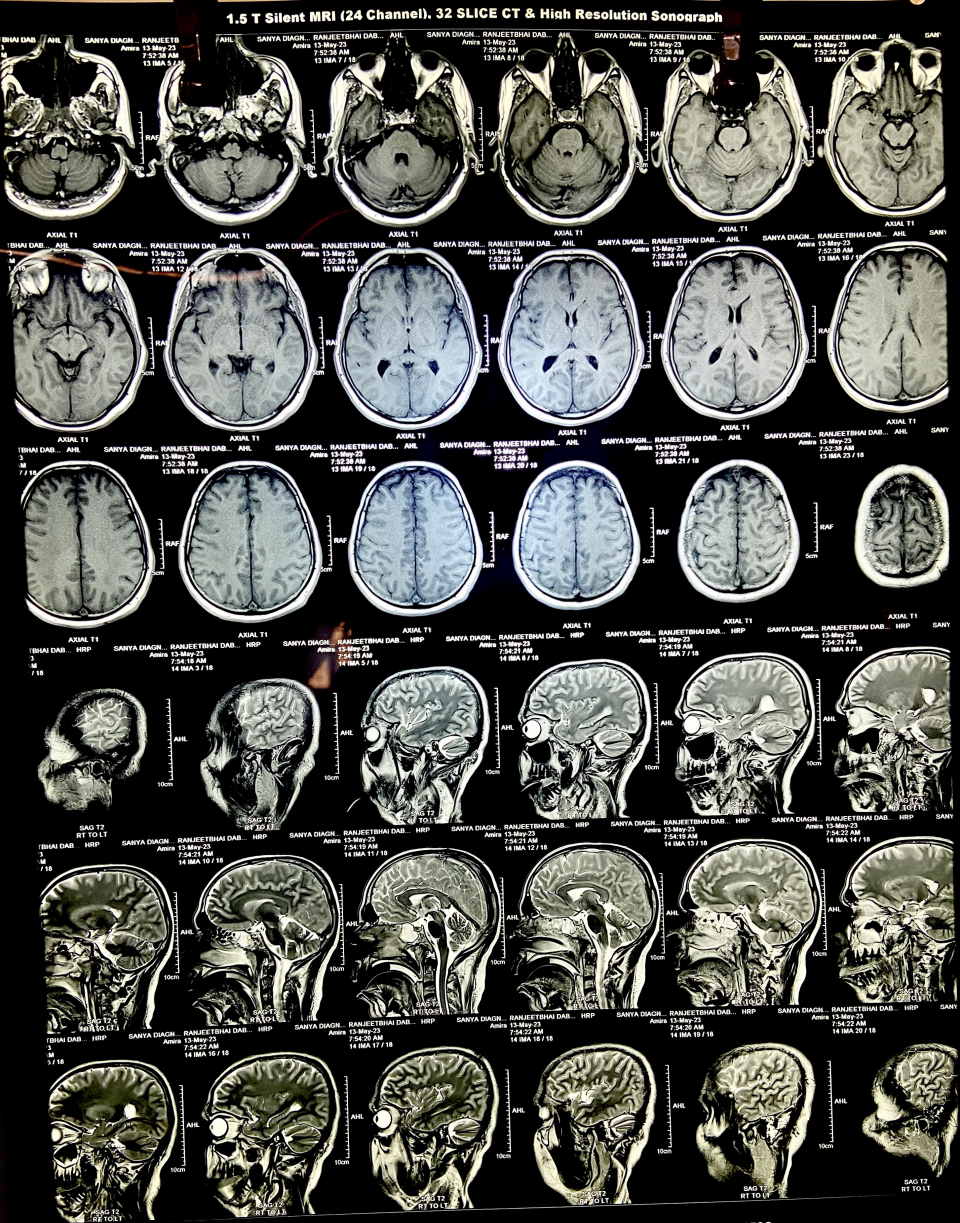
An MRI of the brain, depicting multifocal hypoxic brain injury.
